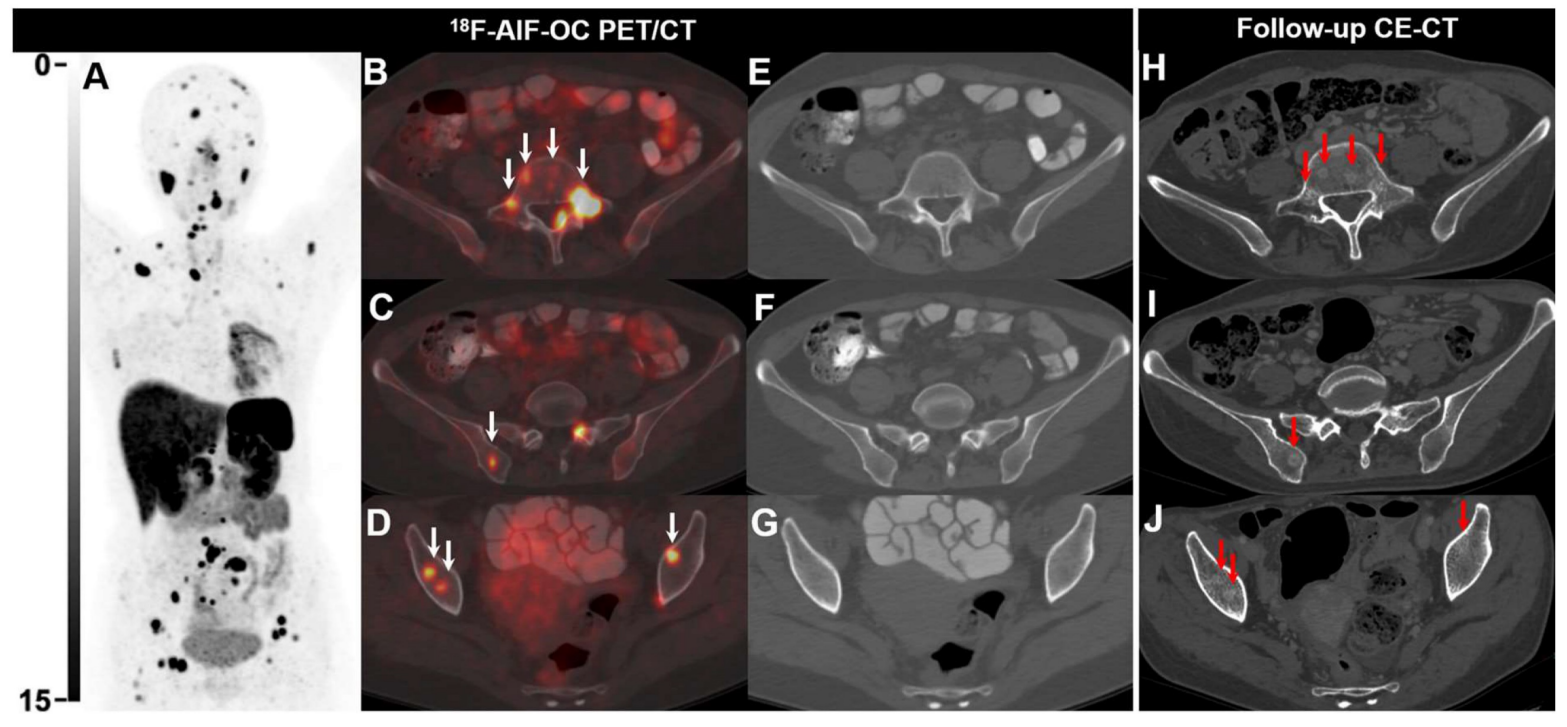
^ 18^F-AlF-OC PET/CT images of a 39-y-old female patient with lung NEN (G2, Ki-67 8%). The patient was previously treated with chemotherapy and radiotherapy. ^18^F-AlF-OC PET/CT was performed for posttreatment restaging and revealed multiple unexpected bone lesions, suggesting progressive disease (A-G). Note that the bone lesions detected on ^18^F-AlF-OC PET/CT were not visible on CT as there were no morphological and density abnormalities (E-G). Subsequently, the patient received somatostatin analogs and targeted therapy (Sulfatinib). 8 months later, follow-up CE-CT revealed multiple osteoblastic changes (red arrows, H-J), corresponding to the bone lesions detected on ^18^F-AlF-OC PET/CT.
